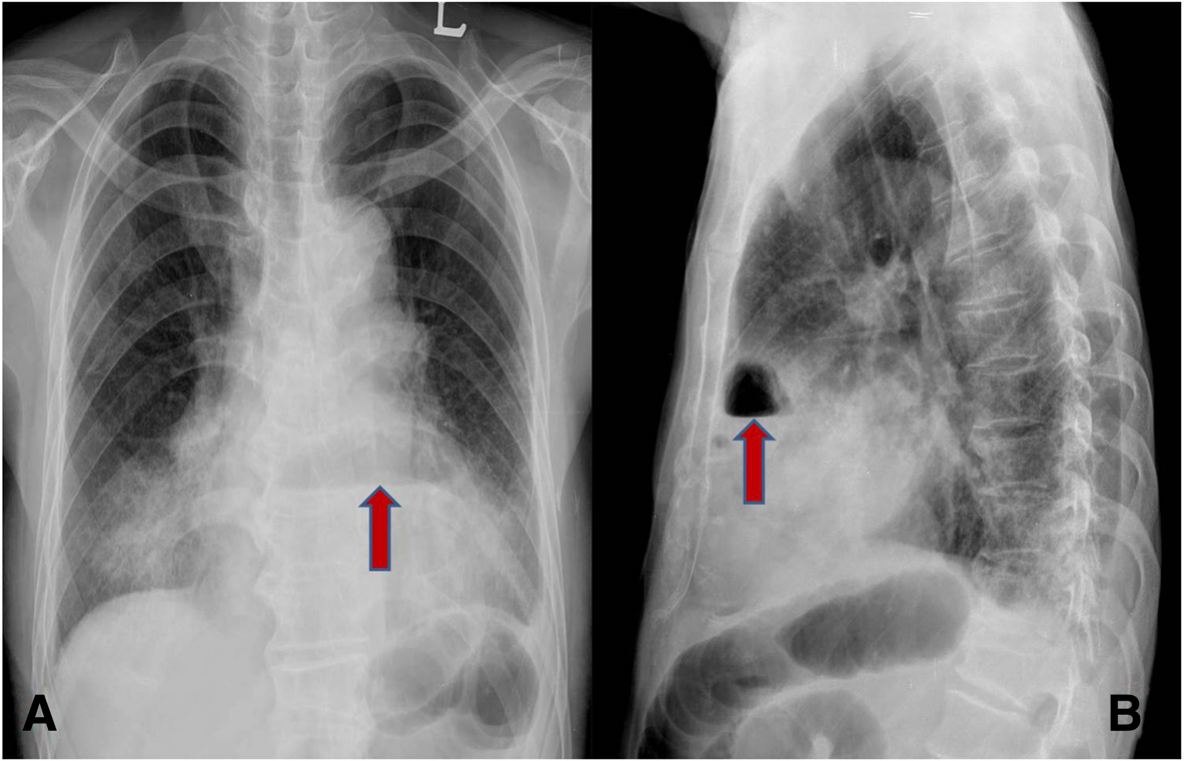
Preoperative chest radiograph. A: Chest PA shows an abnormal air shadow above the diaphragm (arrow). B: Chest lateral view shows an abnormal air shadow under the sternum.
